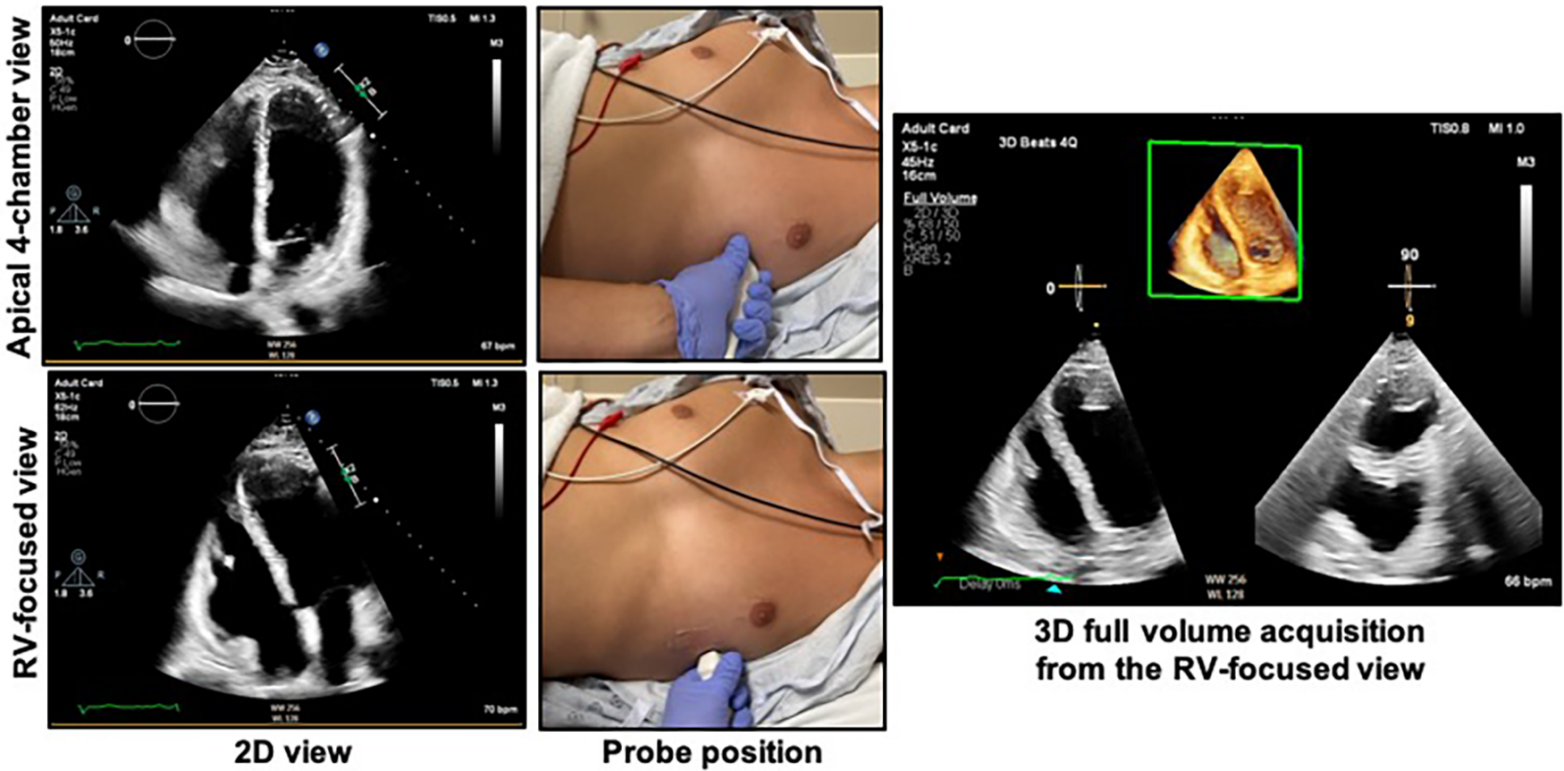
Obtaining the right ventricular focused view. The patient should be in the left lateral decubitus position with the left mid-clavicular, fifth intercostal region (approximate apex of the heart) positioned over the cut-out area of the bed if available. The top right shows the apical 4-chamber view as acquired from the probe position at top middle. The bottom left shows the RV-focused view as acquired from a more lateral location as shown in the bottom middle panel. The 3D dataset should be acquired from the RV-focused view to maximize capture of the RV free wall. See Figure 5 for the characteristics of an optimal 3D dataset. RV, right ventricle.
